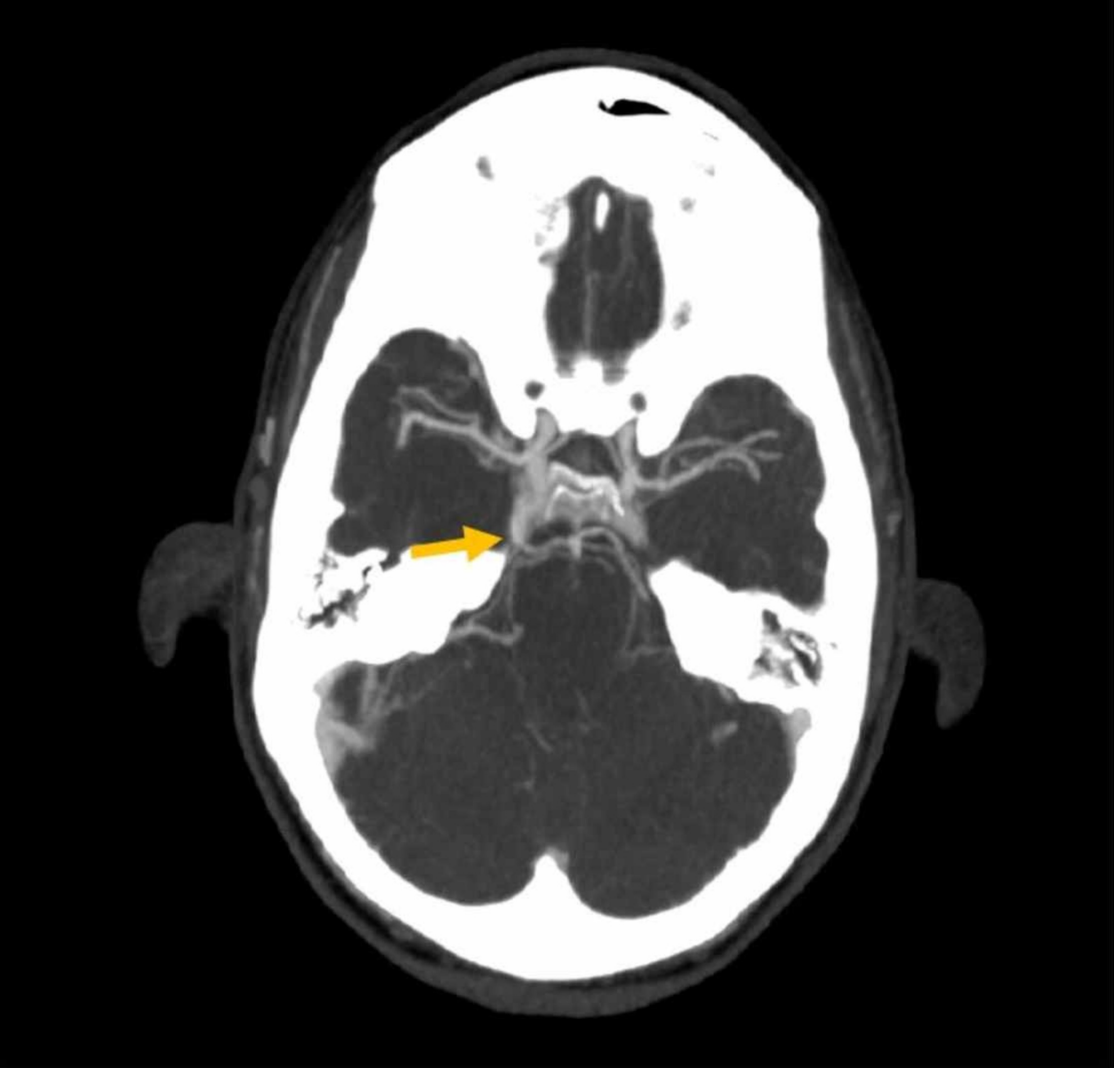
Axial CT angiography of the brain Image displays atypical vessel coursing from the cavernous right internal carotid artery (ICA) to the basilar artery within the prepontine cistern.
